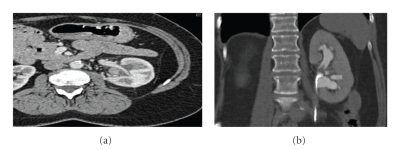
CT Scan findings of fibroepithelial polyp of left kidney.
